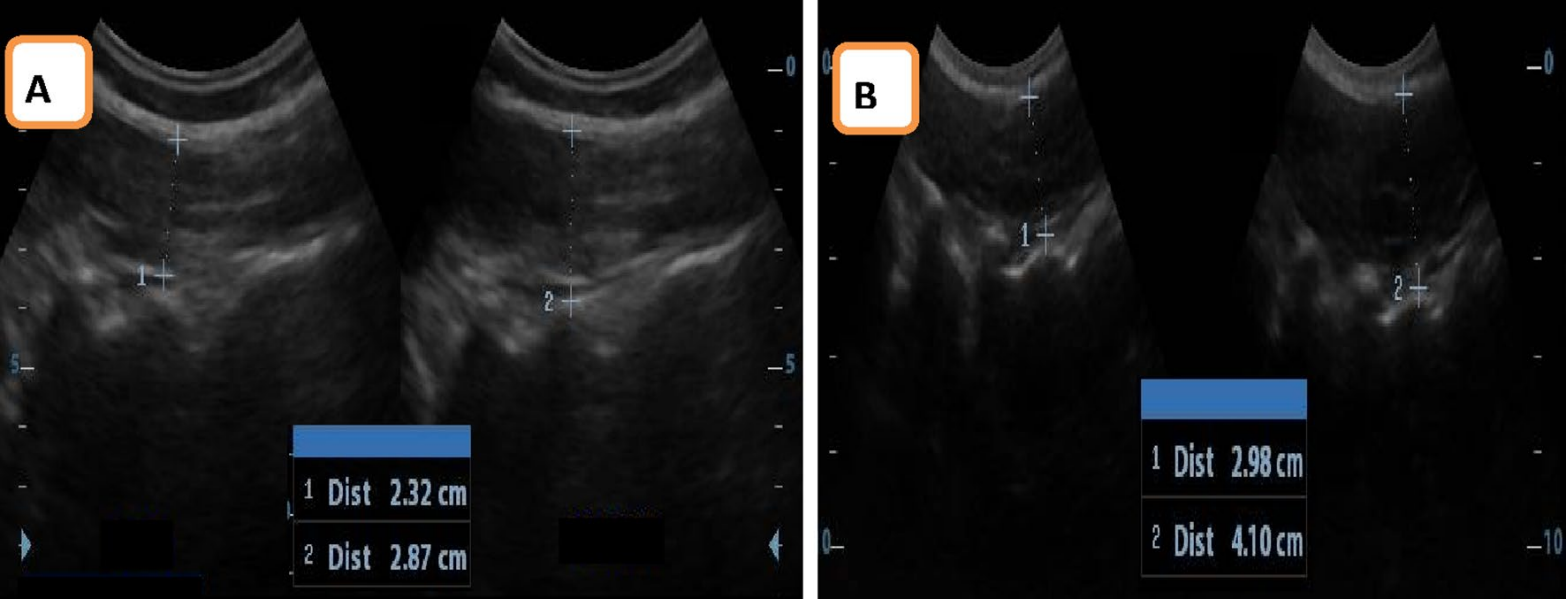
Ultrasound measurement of lumbar multifidus (A) VL group, (B) control group.
